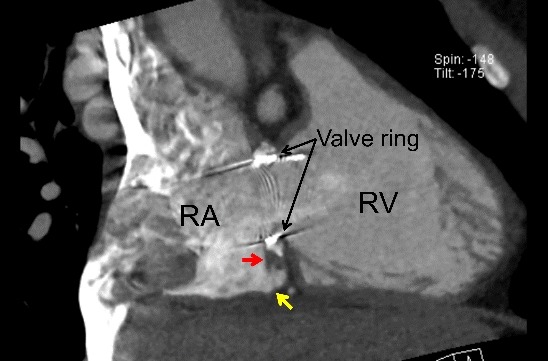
Double oblique computed tomographic image showing the pouch just below the bioprosthetic valve (yellow arrow) and atrial myocardium attached to the valve ring (red arrow). RA, right atrium; RV, right ventricle.
